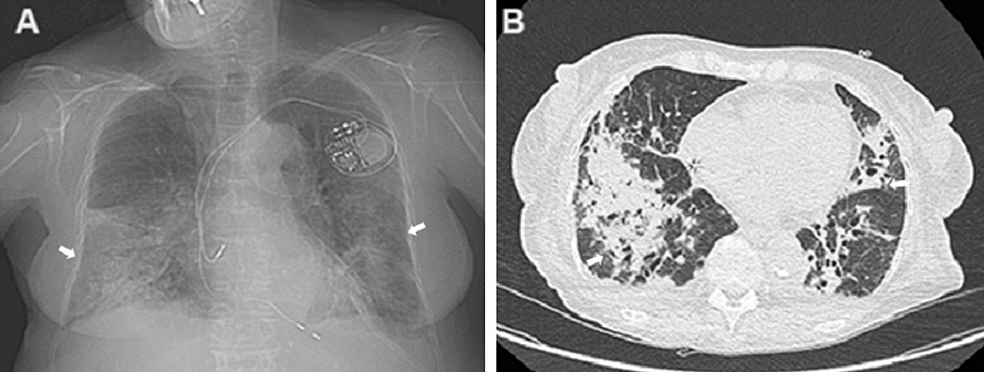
Chest X-ray: bilateral alveolar opacities predominantly at the right base (A); unenhanced chest CT scan, axial section: bilateral consolidations with air bronchogram and indistinct borders (B)
